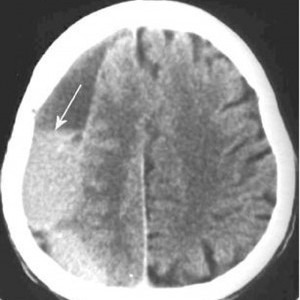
Chronic subdural haematoma with a recent bleeding of right brain hemisphere (CT scan); what should be noted is the mixed, hypo- hyperdense aspect of the haematoma and the erase of the circle movements of the right brain hemisphere as an effect of the compression due to the haematoma
